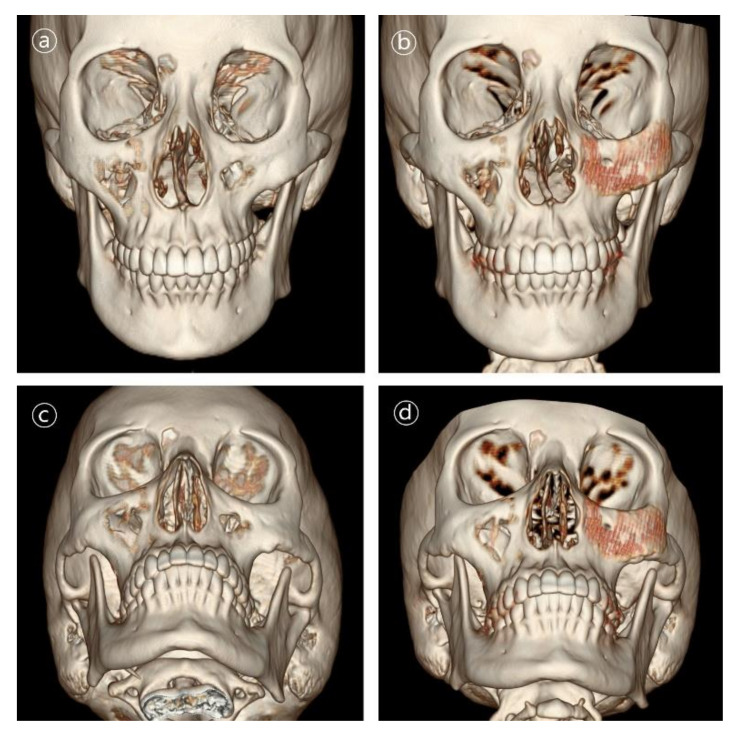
3D CT images in patient #1. Contour and symmetry of left zygomatico-maxillary region was restored with de novo bone formation. (a,b) Pre- and postoperative 6-month frontal view CT images. (c,d) Pre- and postoperative 6-month basal view CT images.
